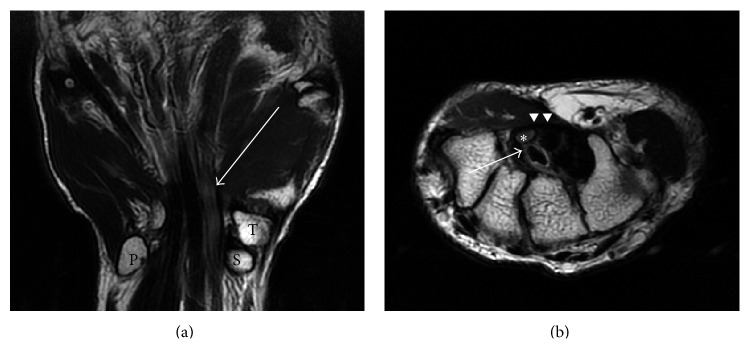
Magnetic resonance imaging of the wrist. (a) T2-weighted coronal image. Edematous lumbrical muscle with synovial proliferation (white arrow) was seen around the flexor tendons. T: trapezium, S: scaphoid, P: pisiform. (b) T2-weighted axial image. The median nerve (*∗*) was compressed between the transverse carpal ligament (white arrow heads) and the edematous synovial tissue (white arrow) around the flexor tendon.
